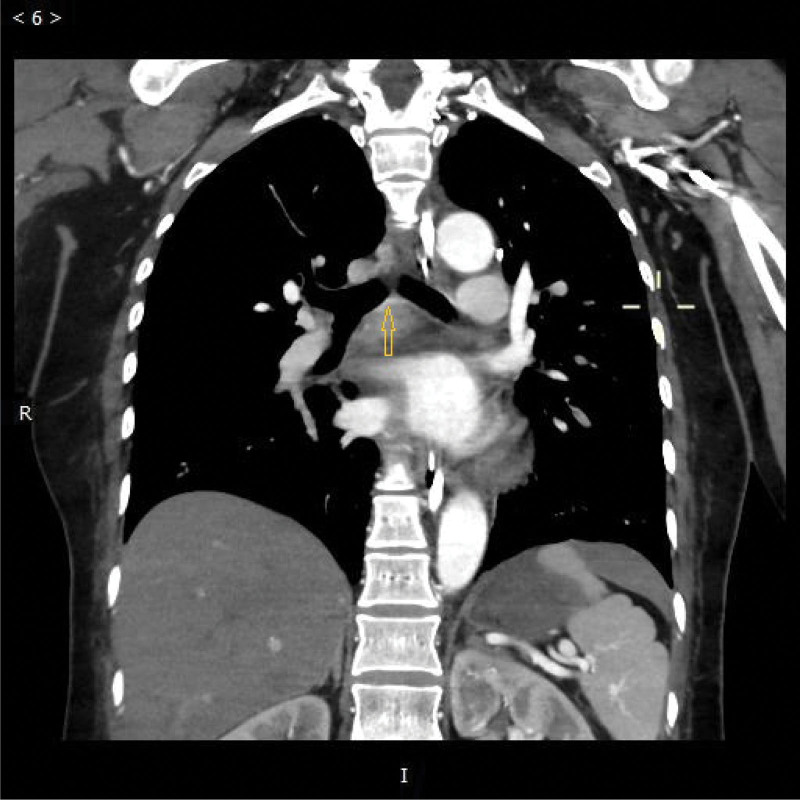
The patient’s abnormal artery around the left principal bronchus of the pulmonary vascular CTA (a, the yellow arrow points to this abnormal artery).
